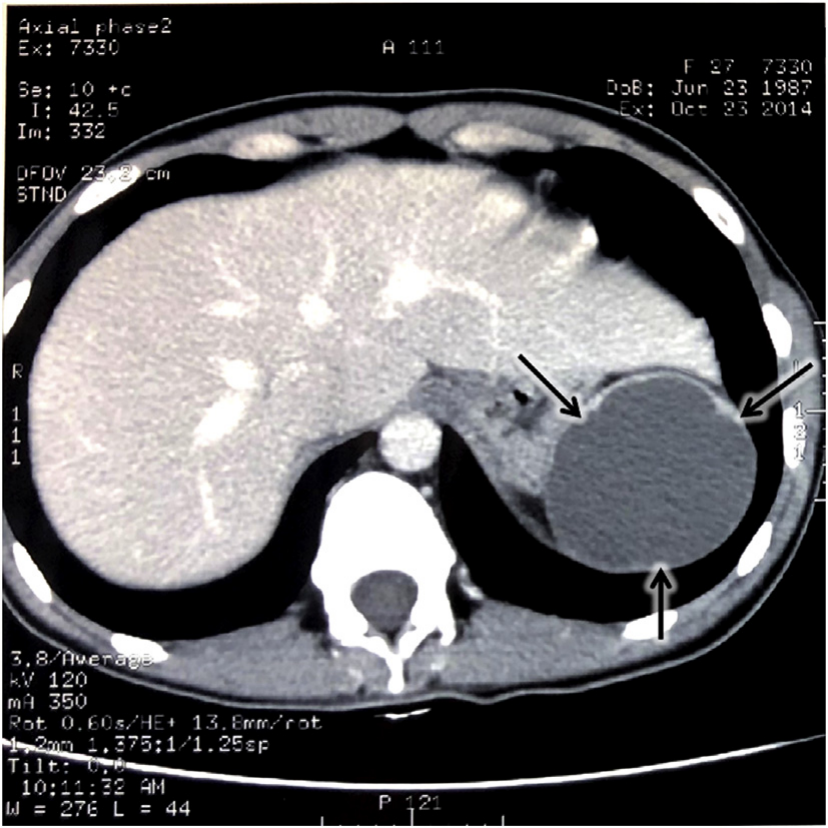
Pre-surgery CT scan of the abdominal region performed on October 23, 2014. Arrows indicate the splenic cyst of 7.5 × 7.5 × 5 cm.
